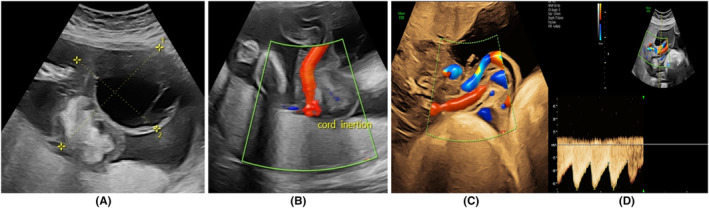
(A, B) Antenatal ultrasound image showing cystic and solid areas. (B) Cord insertion. (c) Note the difference in the calibers of the umbilical arteries arrows. (D) Positive end diastolic flow.
